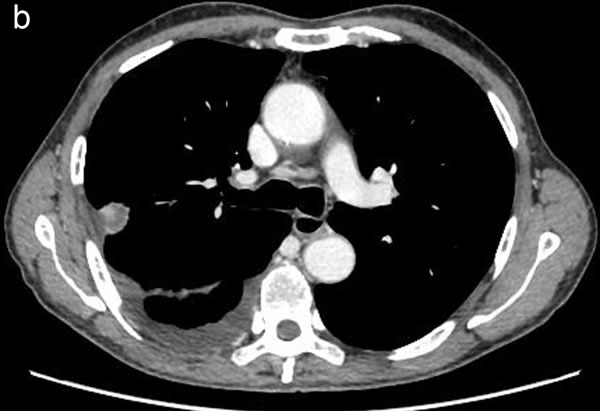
Representative CT images showing homogeneous enhancement (a) and heterogeneous enhancement (b)
